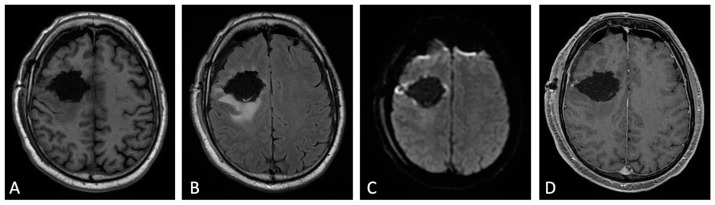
Early post-operative MRI. Axial pre-contrast T1w (A), FLAIR (B), DWI (C), and post-contrast T1w (D). Axial FLAIR (B) shows mild brain edema around the surgical site. No methemoglobin is seen on pre-contrast T1w (A). In DWI (C) a thin rim of hyperintensity (probably due to T2w- shine through effect) around the tumor cavity was attributed to post-operative change. The resection margins are free of enhancement except for a small enhancing area on the lateral aspect of surgical cavity compatible with dilated vein (D). Dural linear contrast enhancement could be seen in immediate post-operative scan (D).
